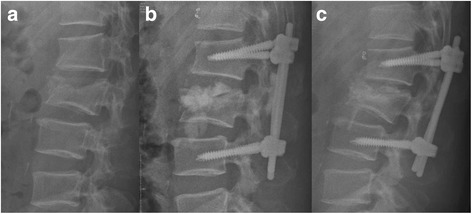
Radiographic image of one patient who received vetebralplasty and short instrumentation. a. Lateral roentgenogram of a 35-year-old male patient showing a L3 burst fracture; the kyphotic angle is 20°. b. Postoperative lateral roentgenogram showing good reduction (the kyphotic angle is 0 degree) through vertebroplasty and short instrumentation with intradiscal and paraspinal leakages 3 days after surgery. c. The kyphotic angle is −3° 12months after surgery and solid anterior fusion (continuous trabeculae of bridging anterior osteophytes without halo signs of screws) was achived. But the kyphotic angle is 12 degree and the rod was bended 12 months after surgery
